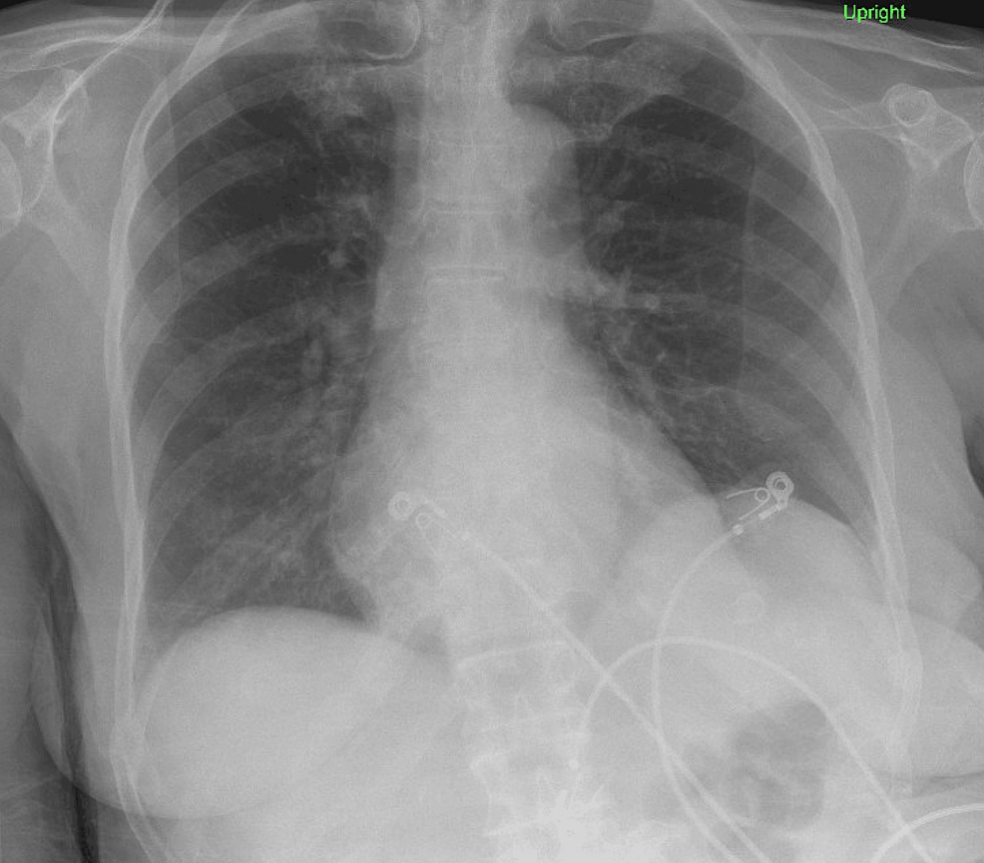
Chest X-ray before daptomycin use
